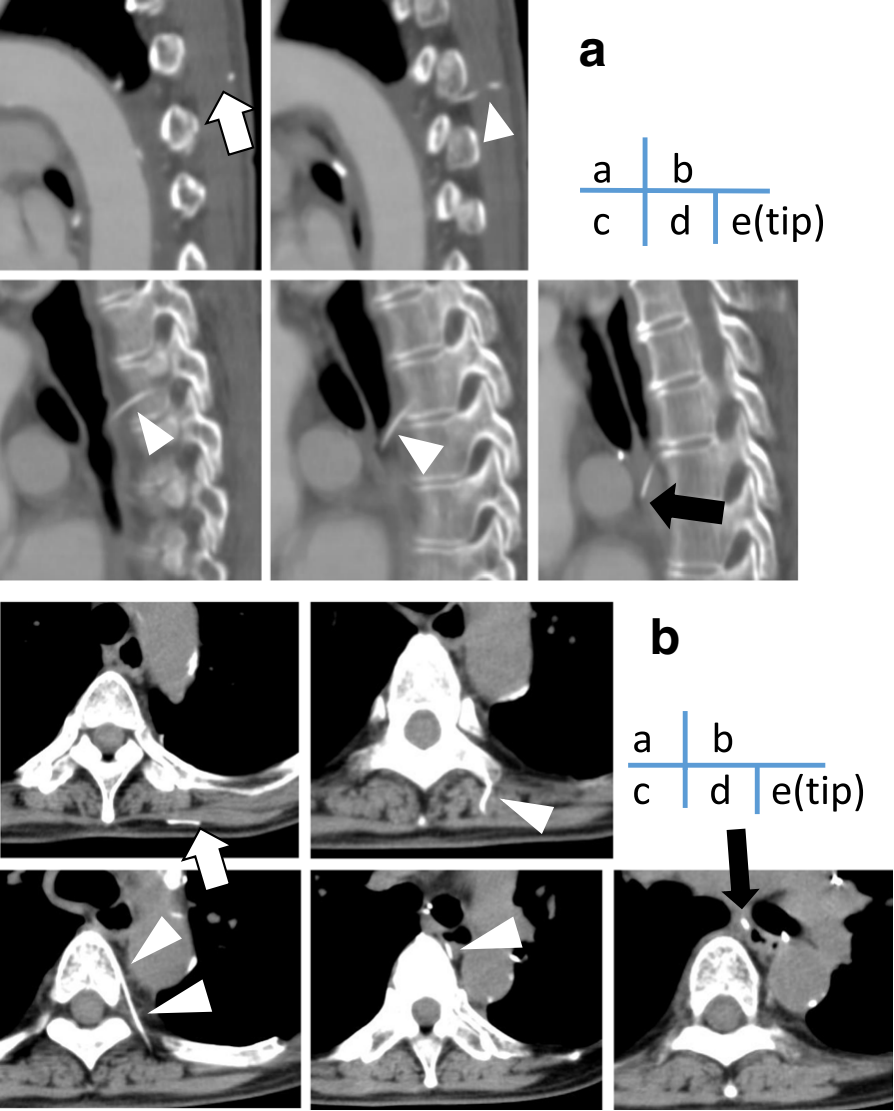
Computed tomography images of the retained catheter fragment (*arrow*). The cut side of the catheter fragment is located at a depth of 6.3 mm from the skin surface (*white arrow*). The tip of the paravertebral catheter (*black arrow*) has been displaced to the posterior mediastinum and is located near the esophagus and trachea, on sagittal views (a) and axial views (b)
